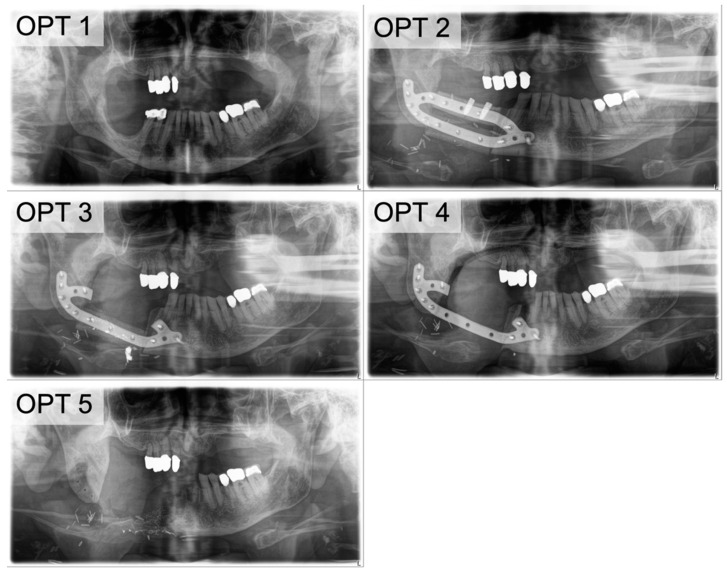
The following OPTs outline the clinical course of TFF in a 76-year-old patient in good general condition who suffered from osseous metastasis of prostate cancer and underwent FFF. (OPT 1) A pathologic fracture occurred after the removal of necrotic bone in the right lower jaw in the setting of bisphosphonate induced MRONJ stage III (treatment with Zoledronacid/Denosumab in prostate cancer) [28]. (OPT 2) Virtual planning of double-barrel fibula was performed. CAD/CAM plate was used for stabilization and the simultaneous insertion of two dental implants was performed. (OPT 3) At 3 weeks post-surgery, the skin paddle was lost. A surgical exploration ended with the removal of an avital distal graft segment and modification of the plate in situ. The vascular pedicle of the remaining FFF still rendered a clear signal in the Doppler ultrasonic probe. (OPT 4) At 16 weeks after surgery, the remaining graft segment also had to be removed in the setting of continued inflammation and the absence of a Doppler signal. (OPT 5) At 22 weeks after surgery: The screws loosened in the anterior mandible segment so that the remaining plate with teeth 32–42 and a bone sequester had to be taken out.
